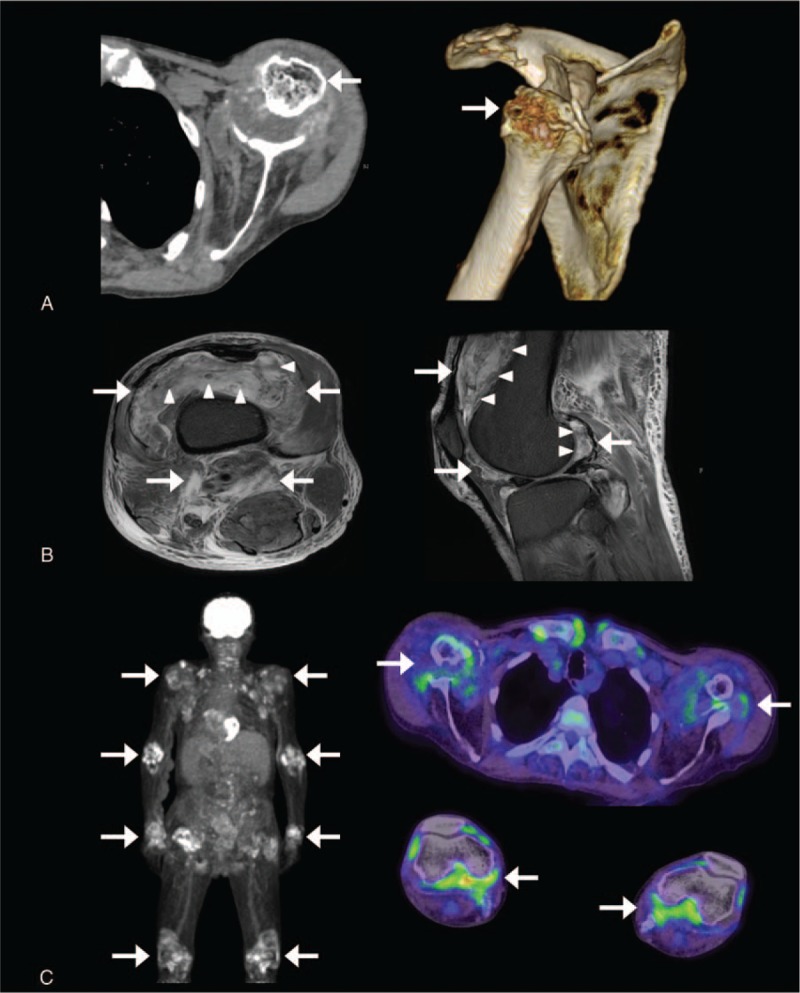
Images of CT, MRI, and 18F-FDG PET of the patients. (A) A representative image of computed tomography of right shoulder. Damaged shoulders (shown by arrows) surrounded by low-density soft tissue was shown. (B) T2-STIR magnetic resonance images of the right knee. A low-intensity area (shown by thick allow) of amyloid deposits and a high-intensity area (shown by thin allow) representing fluid collection. (C) Images of 18F-FDG positron-emission computed tomography. FDG uptake in the large joints was shown with arrows. CT = computed tomography, DRA = dialysis-related amyloidosis, FDG = fluorodeoxyglucose, MRI = magnetic resonance imaging, STIR = T2-weighted short-tau inversion recovery.
